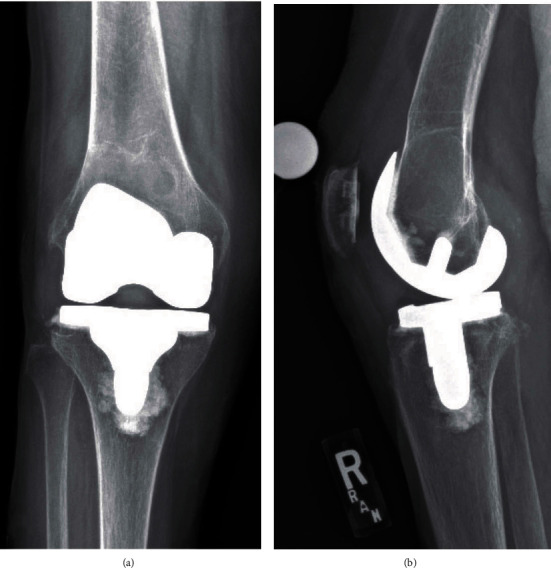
Right knee: (a) AP and (b) lateral radiographs six months later (October 2019).
